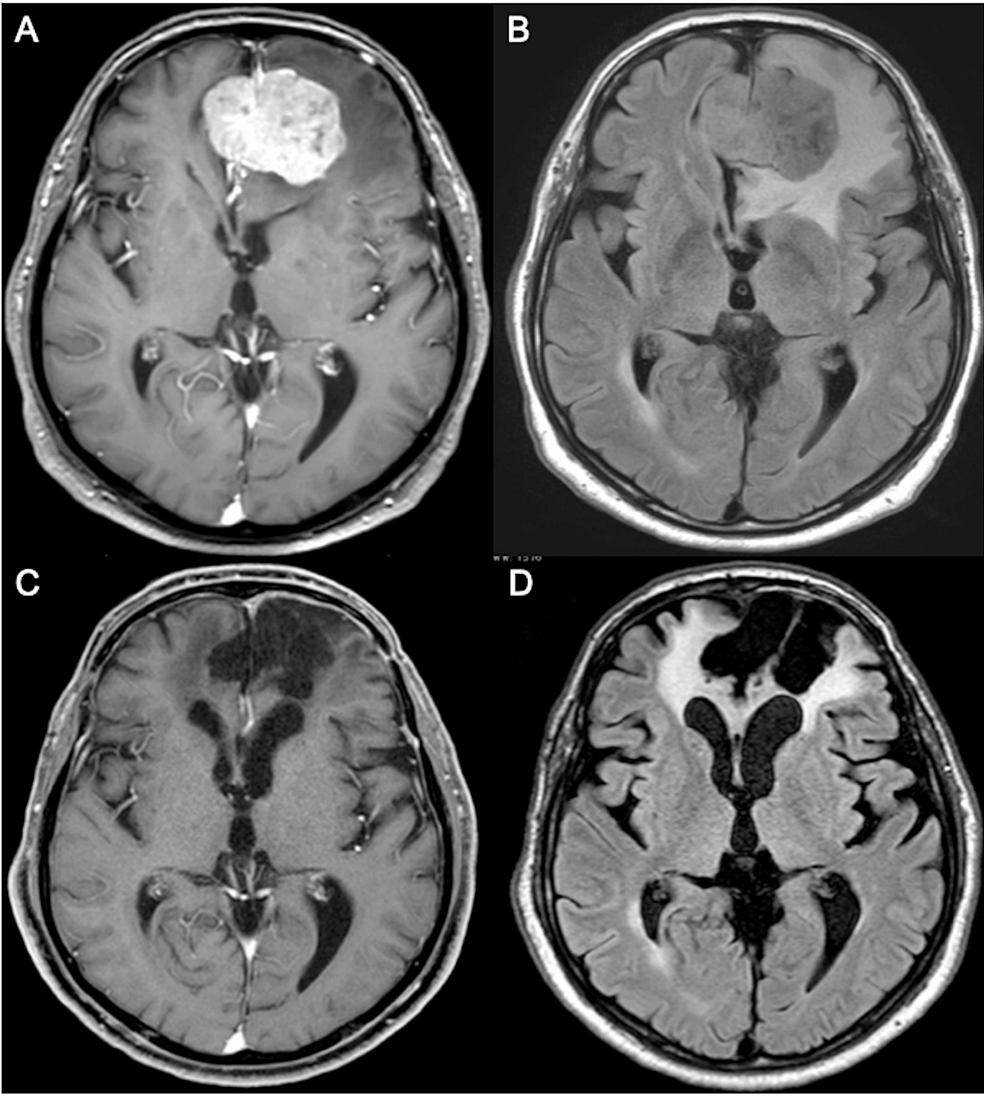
Pre- and postoperative magnetic resonance image of Case 1. (A) Preoperative Gd enhanced axial T1WI. (B) Preoperative axial FLAIR image. (C) Postoperative Gd enhanced axial T1WI. (D) Postoperative axial FLAIR image. Gd: gadolinium; T1WI: T1-weighted image; FLAIR: fluid-attenuated inversion recovery
